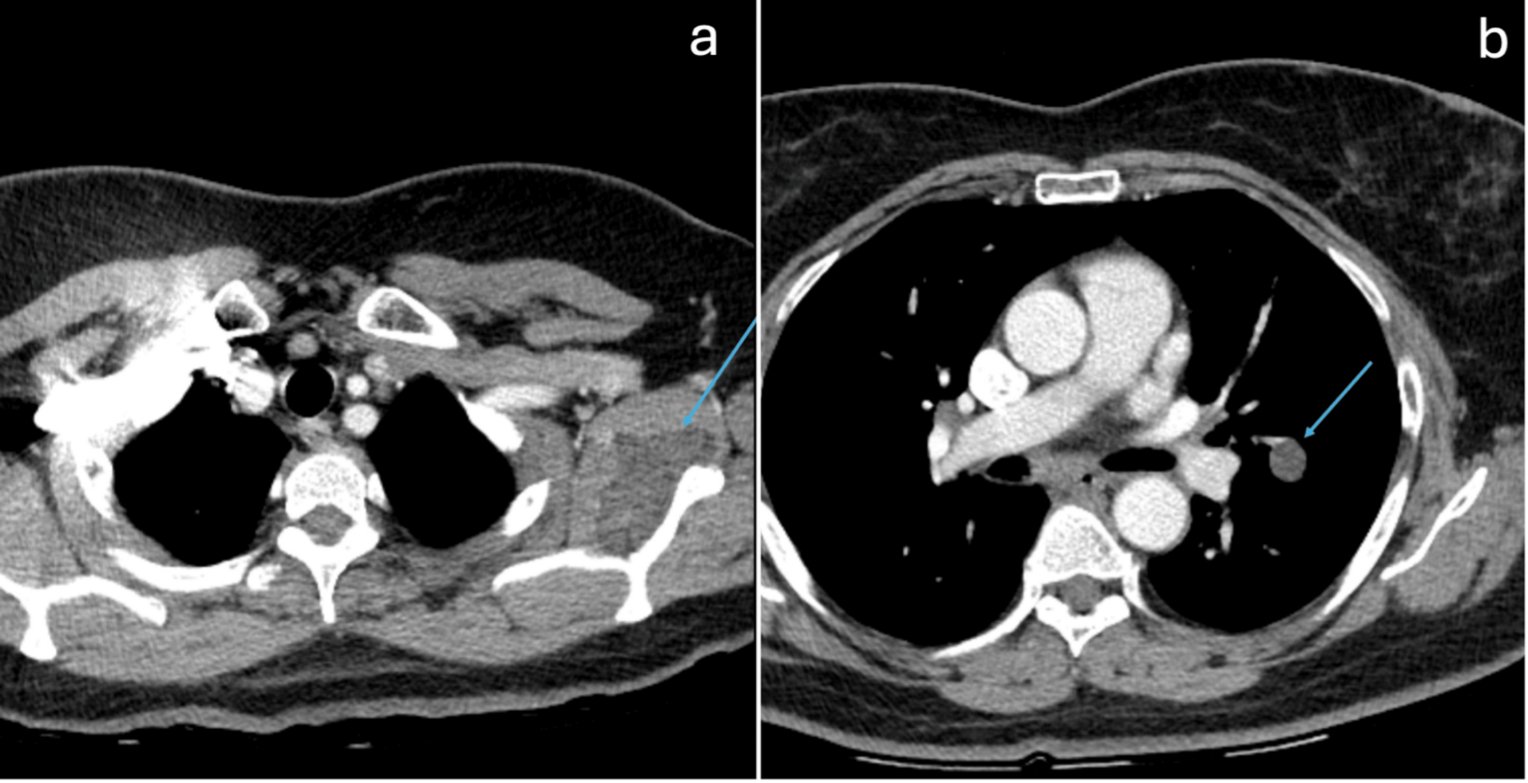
(a) Contrast-enhanced CT of the chest demonstrating a hypoattenuating mass in the left subscapularis muscle that did not measure fat density on CT; (b) Contrast-enhanced CT of the chest demonstrating a hypoattenuating pulmonary nodule. This was also not measuring fat density on CT and was indeterminate and concerning for metastasis at the time.
